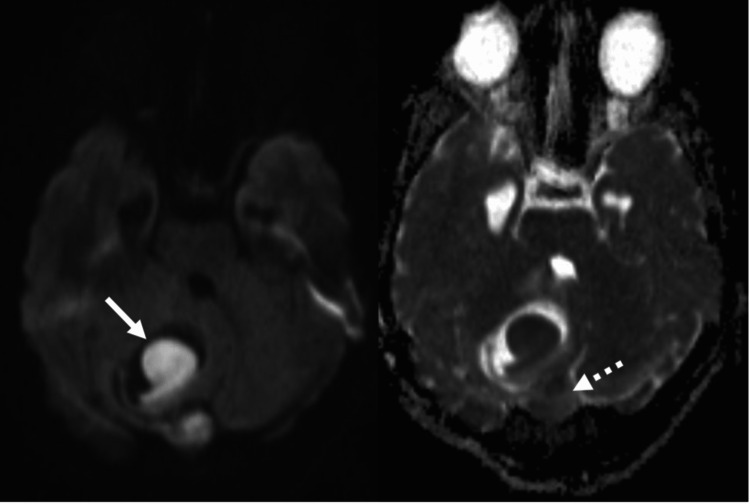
A DWI and ADC map showing a focal central diffusion restriction in the main and daughter cysts, which indicates abscess formation (short and dashed arrows). DWI, diffusion-weighted image; ADC, apparent diffusion coefficient
